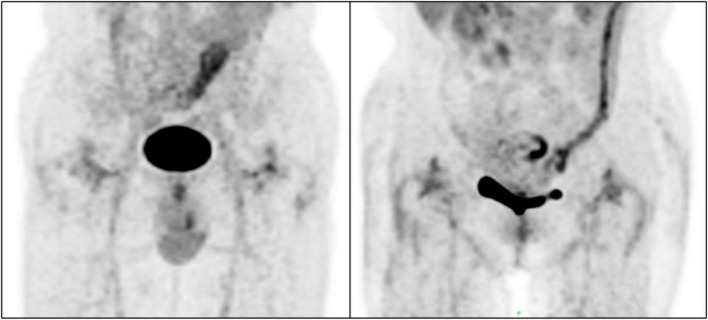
Non-specific uptake of ^18^F-fluorodeoxyglucose (^18^F-FDG) by blood vessels and muscles.
